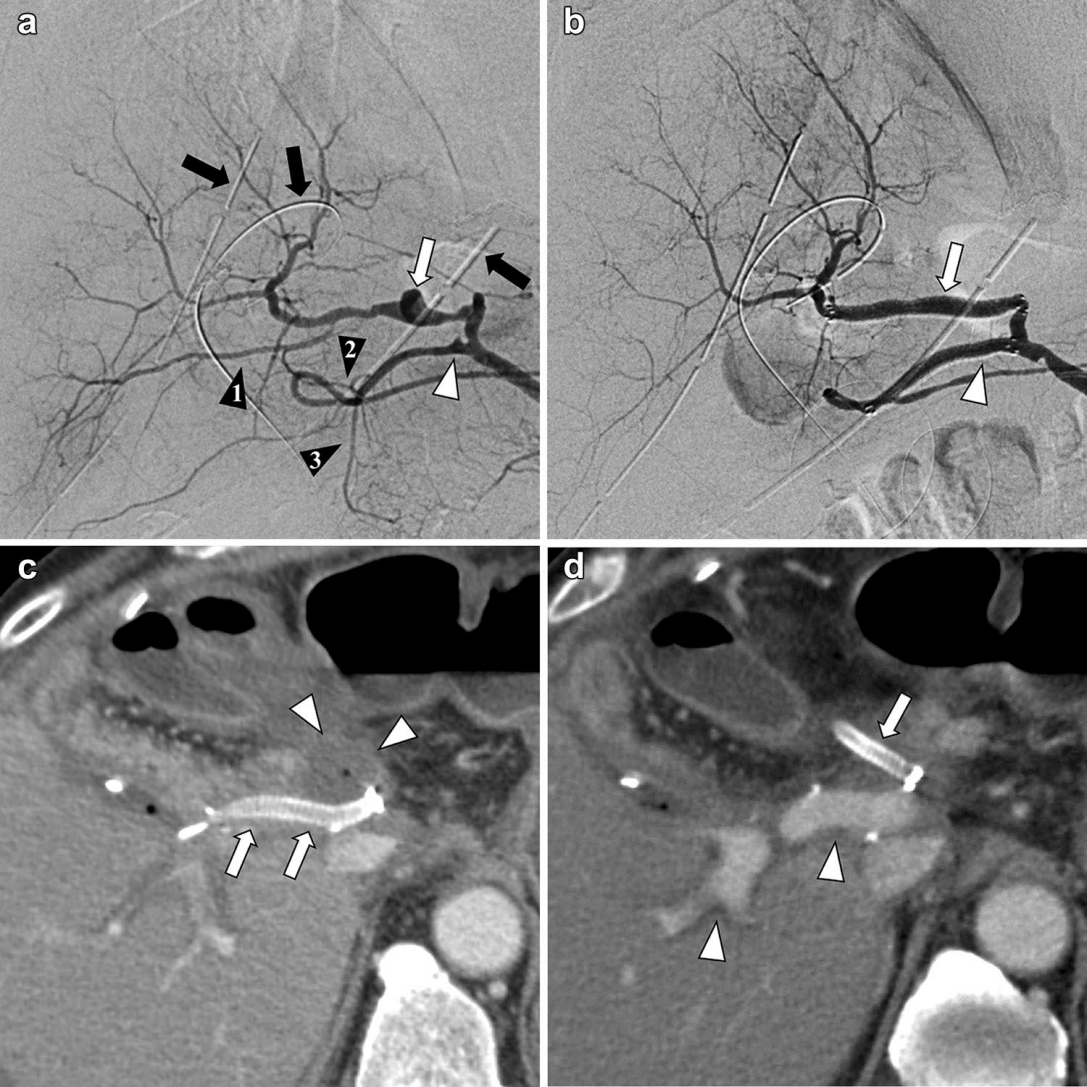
A 78-year-old man with post-left hepatic trisegmentectomy. a The pre-treatment angiogram shows two pseudoaneurysms in the right hepatic artery (RHA) (white arrow) and the gastroduodenal artery (GDA) (white arrowhead). The hepatic artery (A6) (black arrowhead 1) branches from RHA, and posterior superior pancreaticoduodenal artery (PSPDA) (black arrowhead 2) and anterior superior pancreaticoduodenal artery (ASPDA) (black arrowhead 3) branches from GDA. Three drainage tubes (black arrows) are placed in intraperitoneal space. b The angiogram after endovascular therapy with two Viabahn stent-grafts (RHA; 5 mm × 50 mm [white arrow], GDA; 5 mm × 50 mm [white arrowhead]) shows the disappearance of the pseudoaneurysms and a maintained blood flow of peripheral organs. The hepatic artery (A6), PSPDA, and ASPDA are occluded by Viabahn stent-grafts. There are no endoleaks on the angiogram. c Arterial phase of contrast-enhanced computed tomography (CECT) 7 days after treatment indicates the occlusion of the Viabahn stent-graft in RHA (white arrows). There is an abscess surrounding the stent-graft (white arrowheads). d Portal phase of CECT 7 days after treatment indicates the occlusion of the Viabahn stent-grafts in GDA (white arrow). Portal vein (white arrowheads) blood flow is maintained
